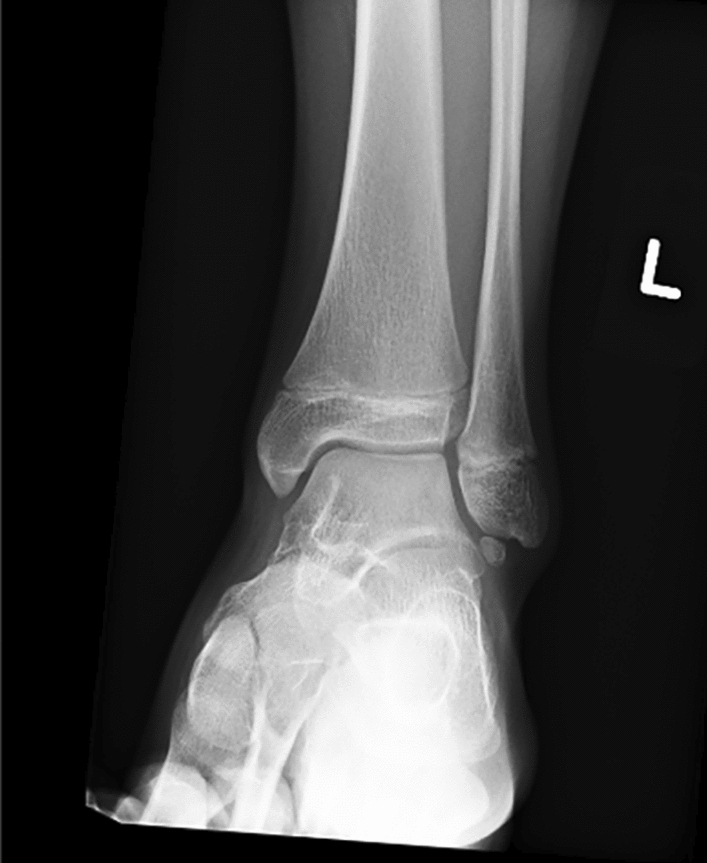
Ankle radiograph with os subfibulare
